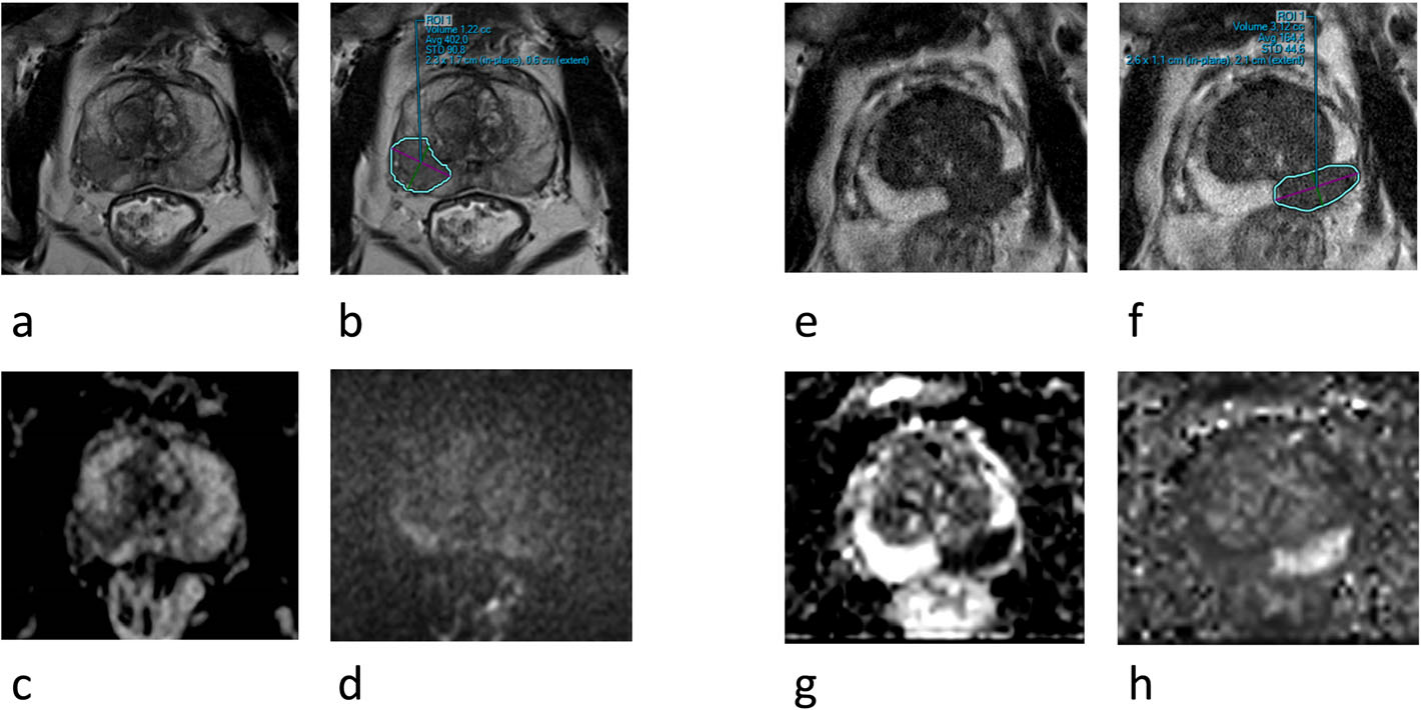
Prostate MRI images from two patients demonstrating poor agreement (images a-d) and good agreement (images e-h). T2 (a and e), T2 with delineated lesion (b and f), ADC (c and g) and high b (d and h) images are shown. In a 64-year old man with PSA of 22 ng/ml (a-d) five radiologist scored a lesion and four did not (fusion biopsies were benign and no PCa has been diagnosed during a 2.5-year follow-up). In a 72-year old man with PSA of 10.5 ng/ml all nine radiologist correctly scored a lesion (GG5 in fusion biopsies)
